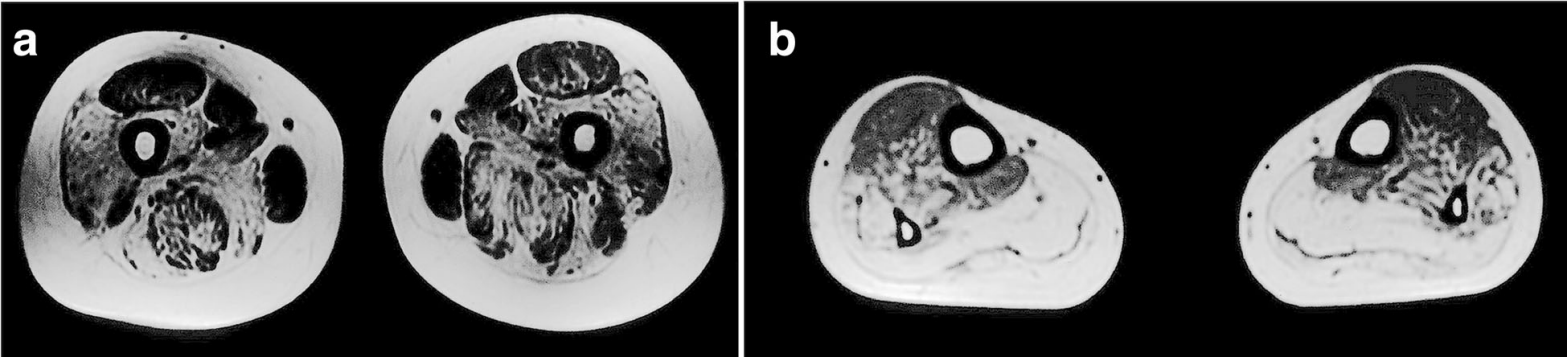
Image and transverse T1-weighted muscle MR image from the patient. MR images demonstrated a typical pattern of muscle involvement (hyperintensities reflect lipomatous alterations). On the thigh level a the vastus intermedius, vastus lateralis, semimembranosus, adductor magnus and long head of the biceps femoris muscles showed moderate lipomatous alterations. On the lower leg level b the soleus, gastrocnemius medialis, and gastrocnemius lateralis muscles show pronounced fatty changes
